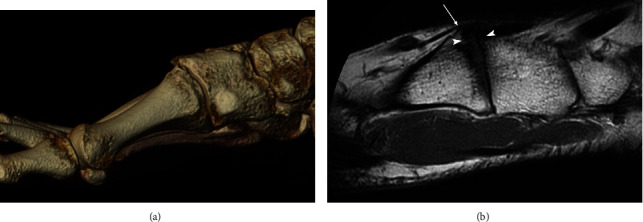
(a) Volume rendered CT image of the medial aspect of the right foot shows the same degenerative disorders as those shown in x-ray images, with a spiky osteophytic deformation on the dorsal aspect of the first tarsometatarsal joint. (b) Sagittal T1-weighted MRI image of the right foot shows a dorsal joint space narrowing (arrowheads), hypo T1 subchondral sclerosis and osteophyte formation (arrow).
